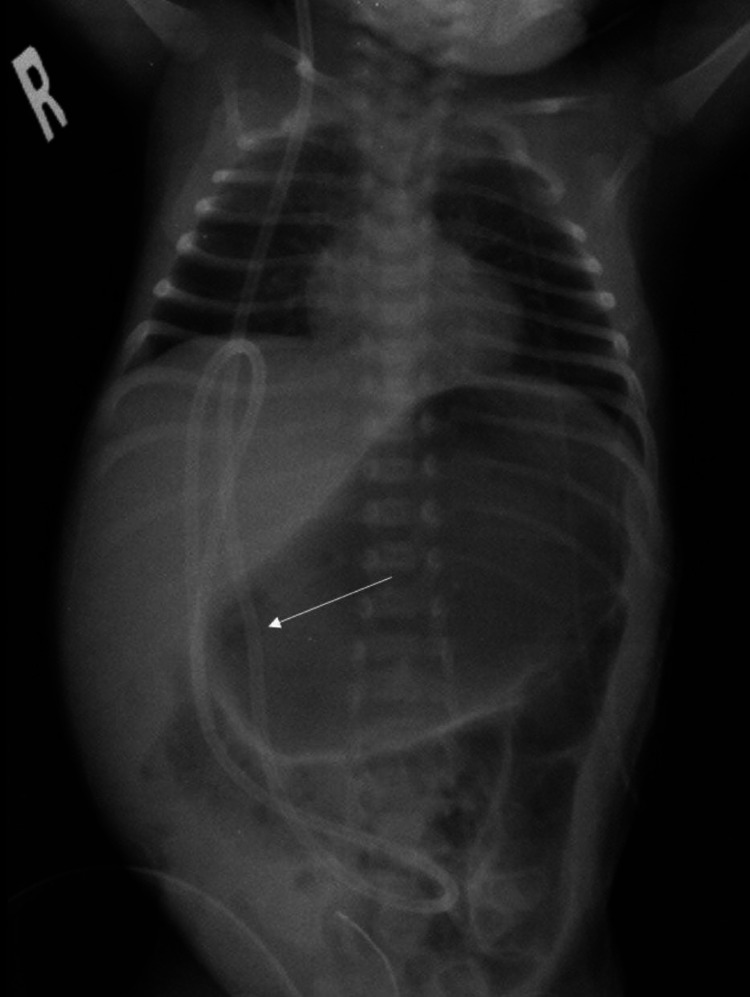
After the first operation, chest X-ray shows the extension of the catheter from the neck toward the peritoneal cavity.
